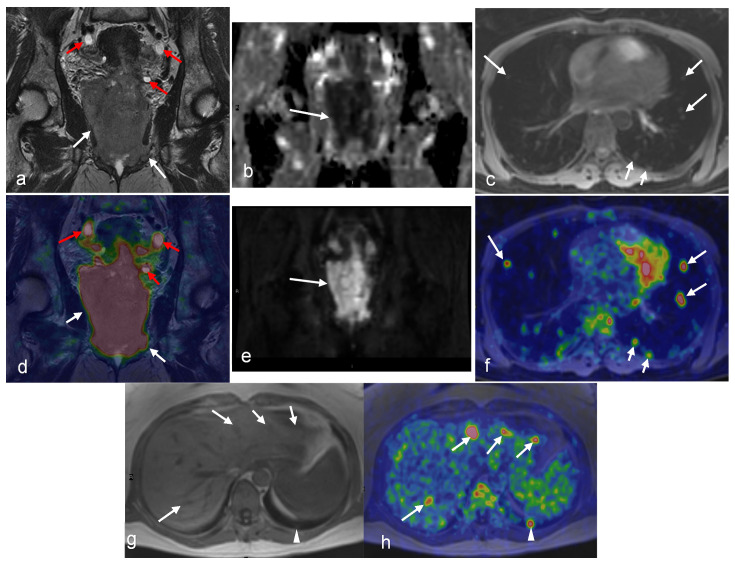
A 49-year-old woman with stage IVB cervical cancer. Both the coronal T2-weighted image (a) and fused PET/MRI (d) demonstrate the tumor with parametrial involvement and almost the entire vaginal vault (white arrows in (a,d)). There is also involvement of the bladder, ovaries, and proximal ureters (not imaged). Bilateral hydronephrosis is partially visualized (red arrows in (a) and (d)). The mass demonstrates diffusion restriction on the ADC map (arrow in (b)) and bright signal on the DWI (arrow in (e)). Axial Dixon water MRI (c) and axial-fused PET/MRI (f) show lung metastasis (arrows). Liver metastasis (arrows) and additional lung metastasis (arrowhead) are demonstrated in axial Dixon in-phase MRI (g) with high FDG uptakes on axial-fused PET/MRI (h). (Courtesy of Elisabeth Hedlund, MD, Håkan Ahlström, MD, and Björg Jónsdóttir, MD, PhD, Uppsala University, Uppsala, Sweden).
